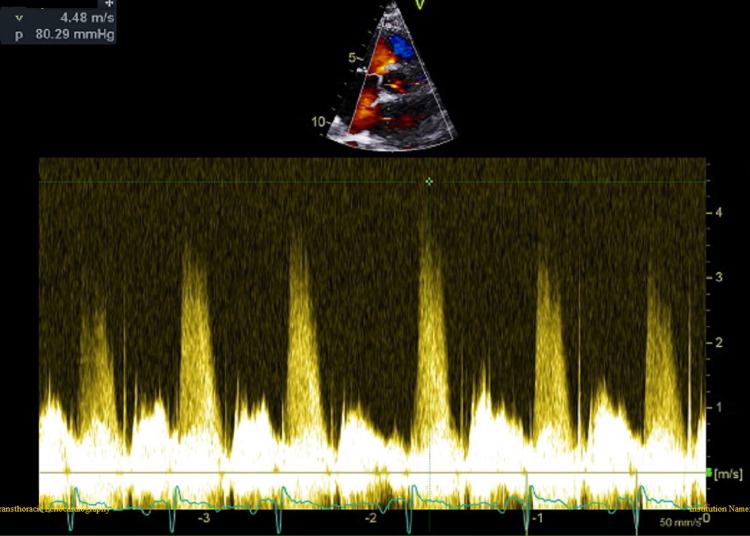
Continuous wave Doppler aligned with ventricular septal defect showed an LV-RV gradient of 80 mmHg. LV: left ventricle; RV: right ventricle.
